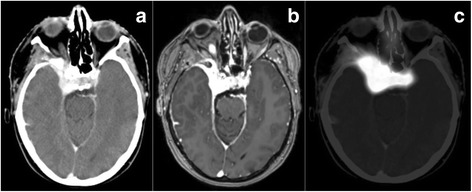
Imaging of a typical skull base meningioma on a contrast-enhanced CT, b contrast-enhanced MRI and c
